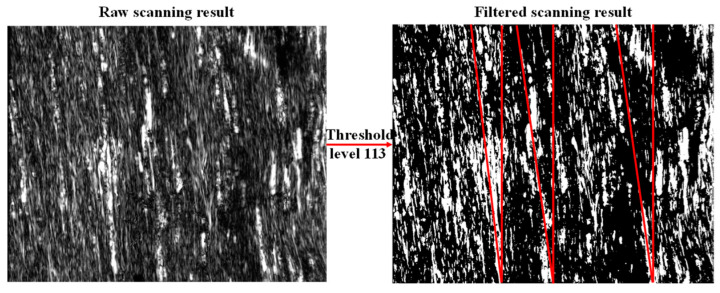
Ultrasound C-scan imaging of UD.
